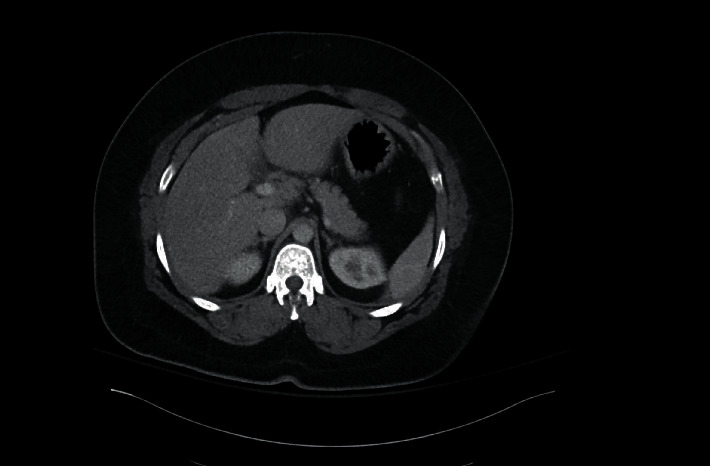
35-Year-old female with biliary colic concerning for acute cholecystitis. Findings: contrast-enhanced CT images of the abdomen in the portal venous phase in the sagittal (1a), coronal (1b), and axial (1c) planes demonstrate empty gallbladder fossa. Technique: axial CT with sagittal and coronal reconstructions, 158 mA, 120 kV, 3 mm slice thickness, and 80 ml Omnipaque 350 intravenous contrast.
